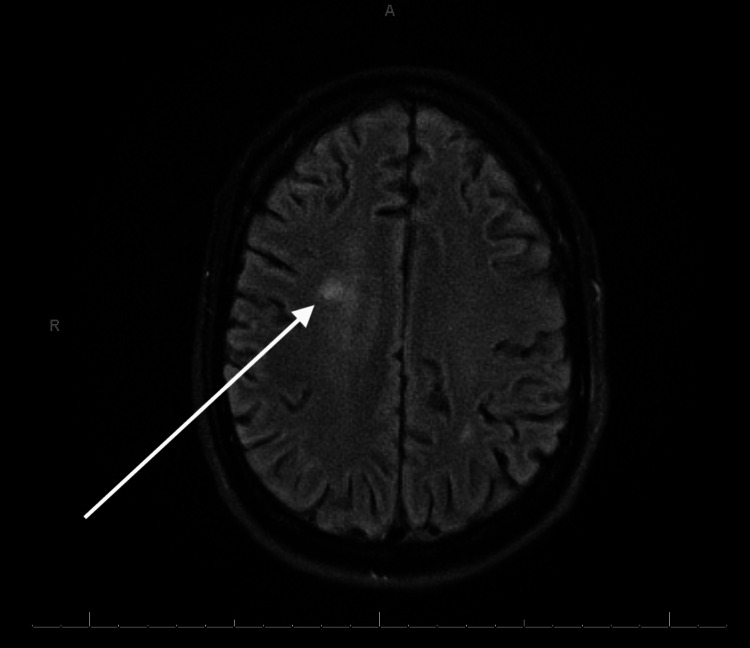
MRI of the brain revealing small acute cortical infarct
